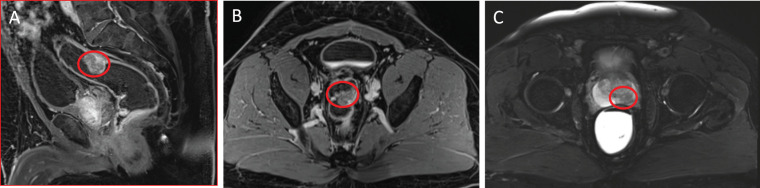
Sagittal (A) and axial (B) T1 MRI images with contrast showing a hypervascular mass involving the posterior rectum without evidence of extension into the perirectal fat (a T2 lesion). (C) Axial T2 MRI image showing a hypointense lesion in the posteromedial aspect of the peripheral zone of the mid prostate gland (organ confined and without extracapsular extension). Abbreviation: MRI, magnetic resonance imaging.
